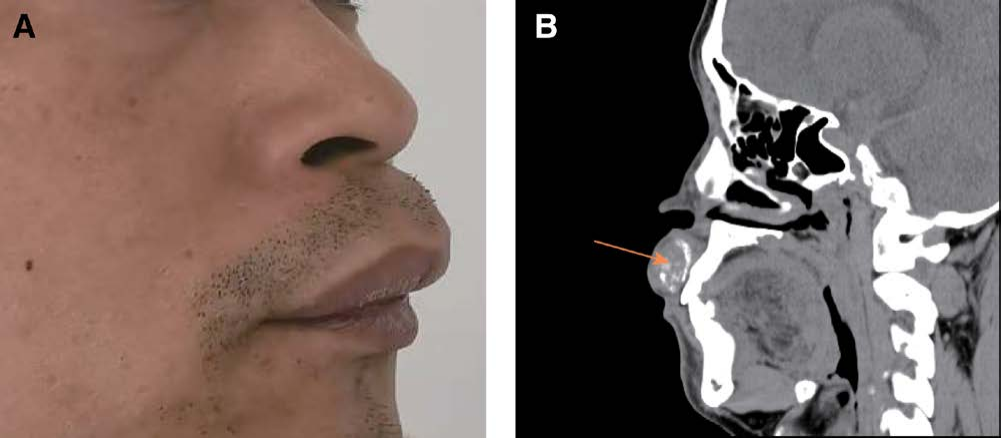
Preoperative clinical photograph and CT findings. (A) Lateral facial view showing swelling of the upper lip. (B) Axial CT image demonstrating a well-defined upper lip lesion with multiple internal calcifications. CT = computed tomography.
